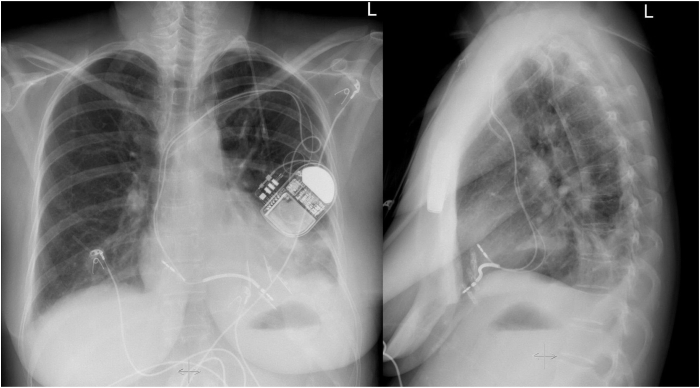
Postprocedural radiograph.
